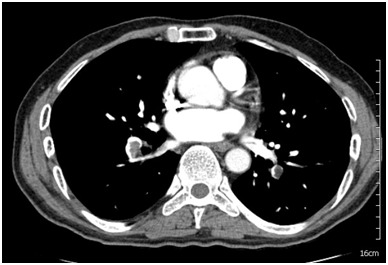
Spiral chest CT scan demonstrates bilateral filling defects of pulmonary arteries.
